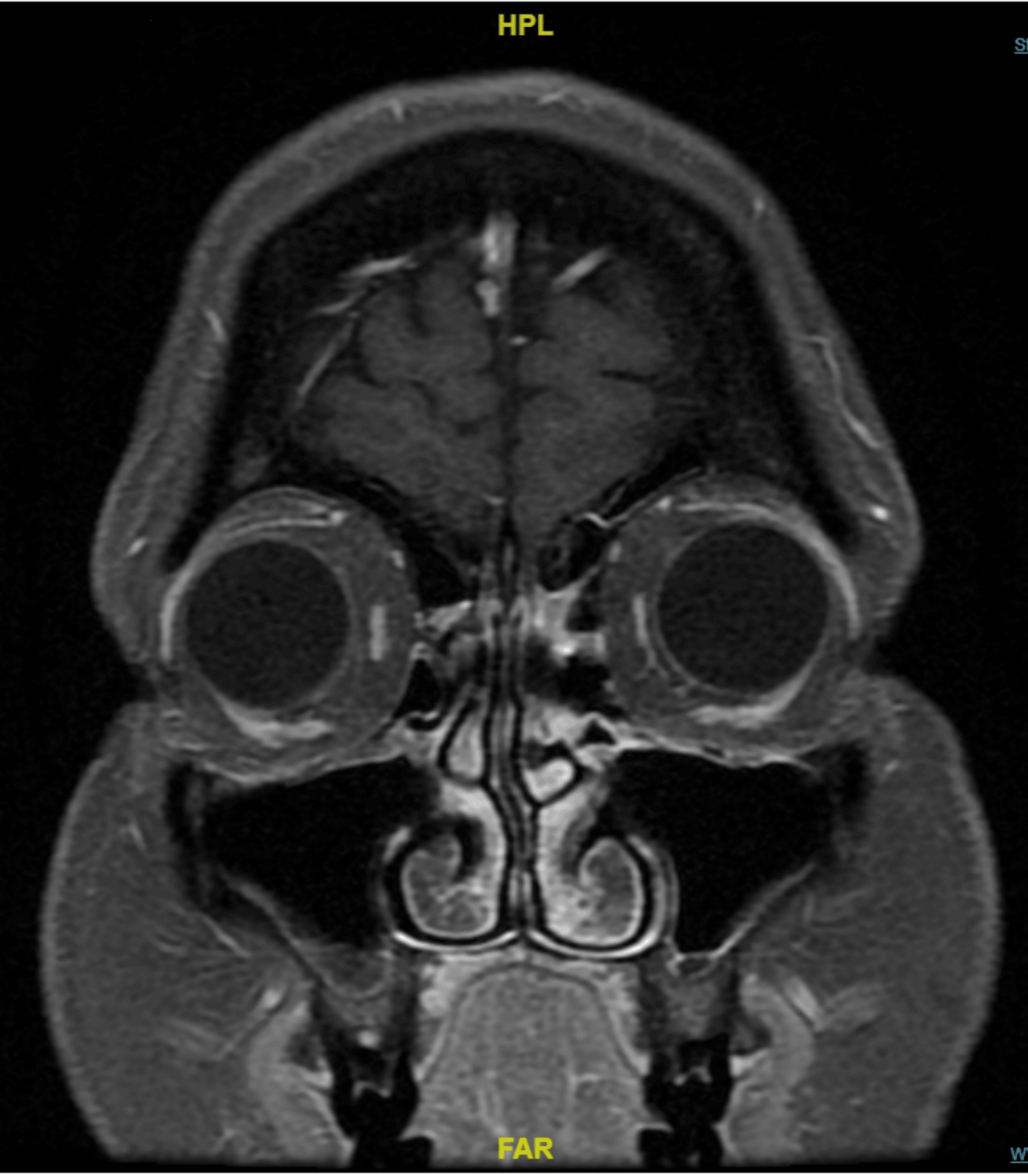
MRI scan The orbits demonstrate resolution of the inflammatory process previously identified in the left superior orbit. The structures now have a symmetric, physiological appearance. The globes are intact. No preseptal abnormalities are found.
